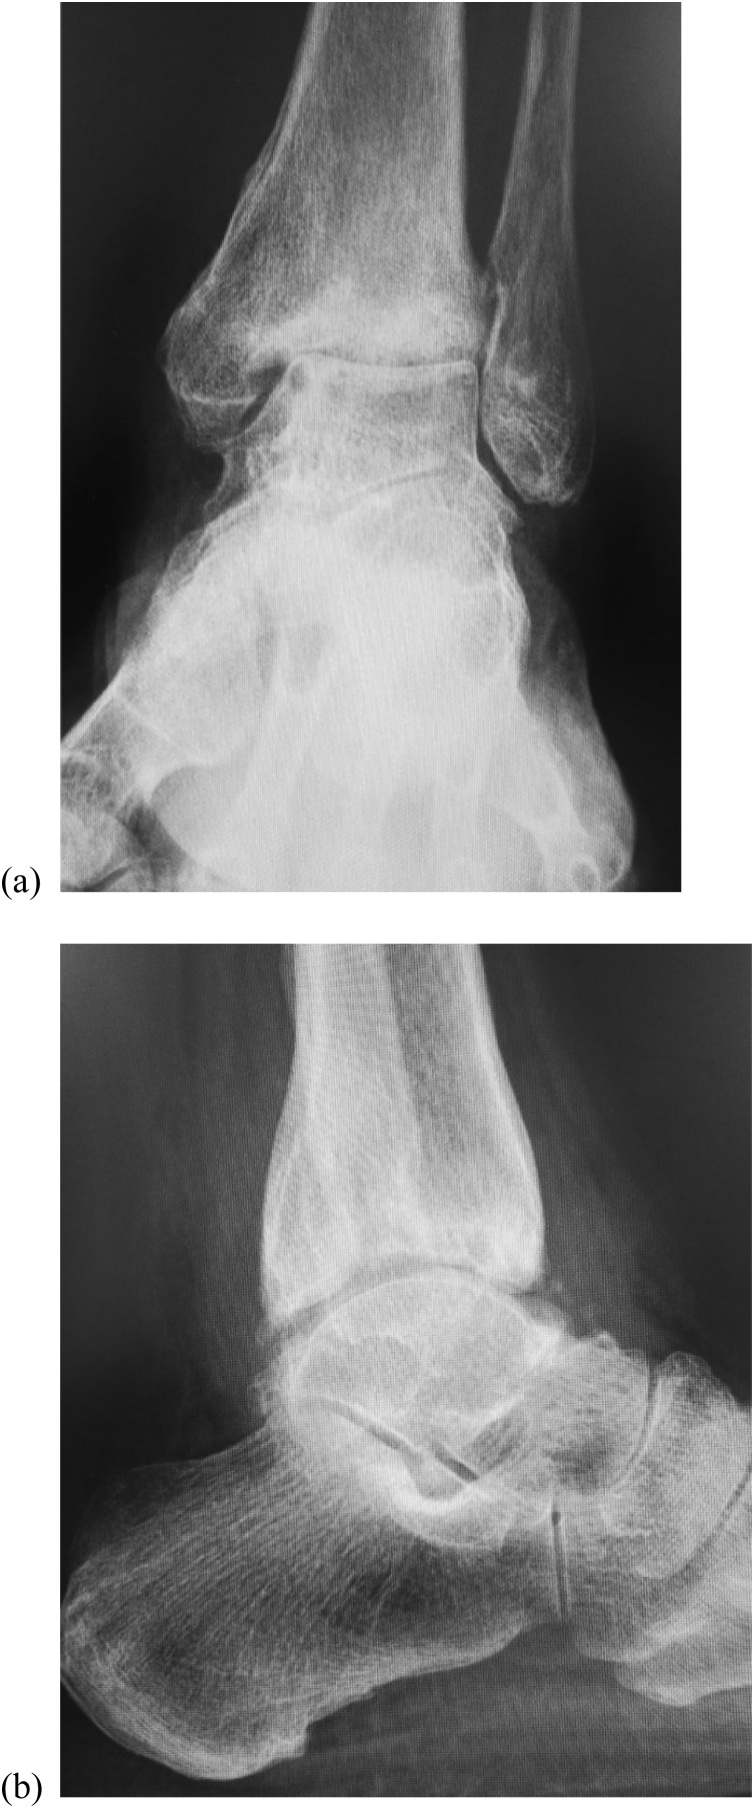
Preoperative plain radiography images. Images showing severe tibiotalar joint space narrowing, indicating osteoarthritic change in the standing position on (a) anterior-posterior view and (b) lateral view.
